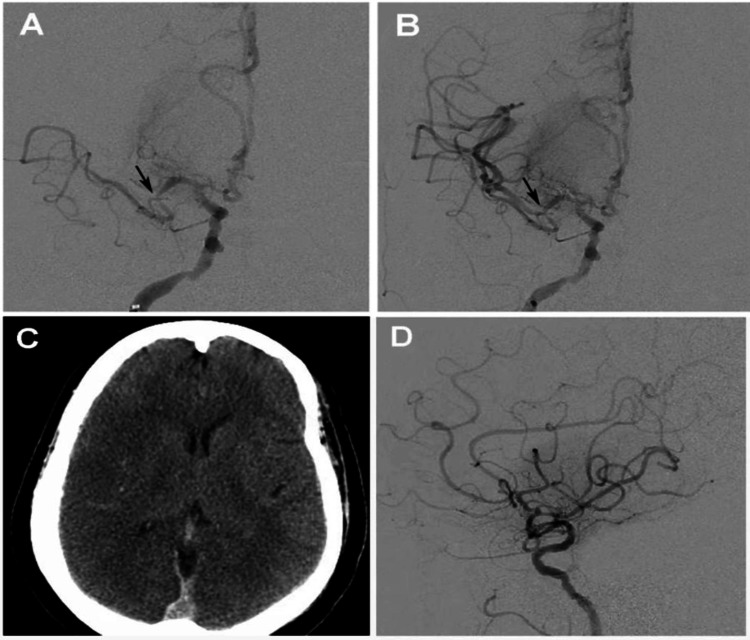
Middle Cerebral Artery Occlusion and Revascularization A) Pre-thrombectomy right internal carotid artery cerebral digital subtraction angiography (DSA) angiogram demonstrating a cutoff at the middle cerebral artery (MCA) bifurcation (black arrow) with only the anterior temporal artery filling distally. B) Post-thrombectomy, AP view shows successful revascularization of the MCA territory but notable moderate focal stenosis at the prior occlusion point (black arrow) suggestive of underlying atherosclerotic disease. C) CT brain without contrast performed 48 hours after intervention demonstrating hypodense right MCA territory cortex with loss of grey-white differentiation and right MCA territory hypodensity (edema) consistent with large volume MCA territory infarct. D) Lateral view after revascularization demonstrating flow in the superior and inferior division but delay in filling distally compared to anterior cerebral artery (ACA) territory felt to be related to infarct core.
